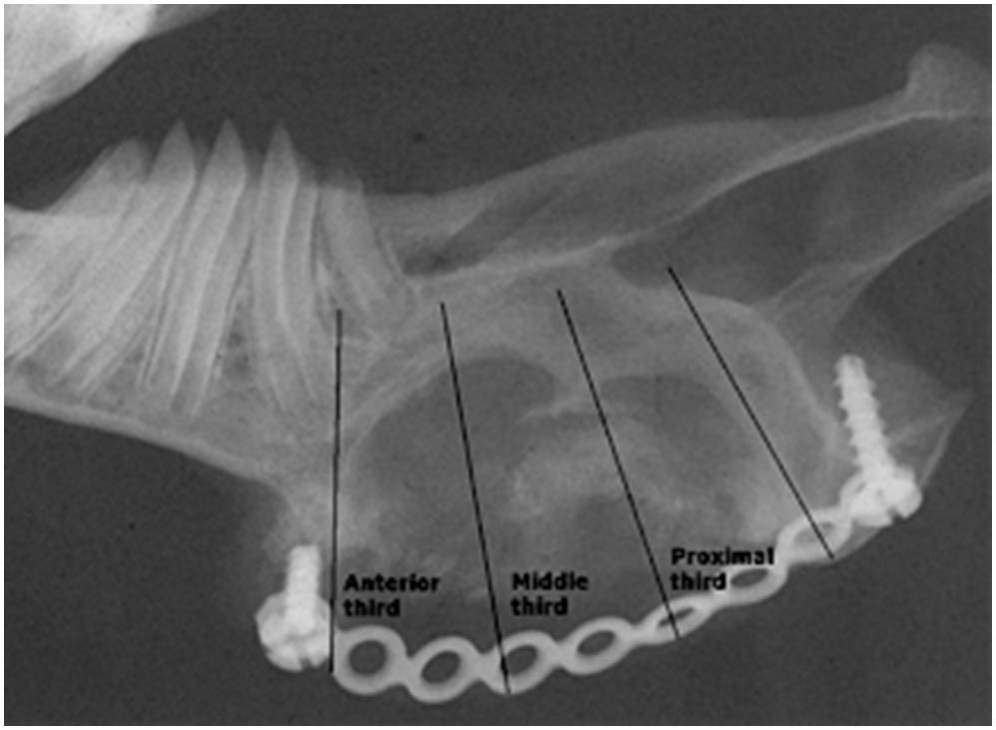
Radiographic image of the ramus of the mandible three months following surgery, showing the anatomical subdivision of the radiograph into anterior, middle, and posterior thirds for qualitative assessment.
